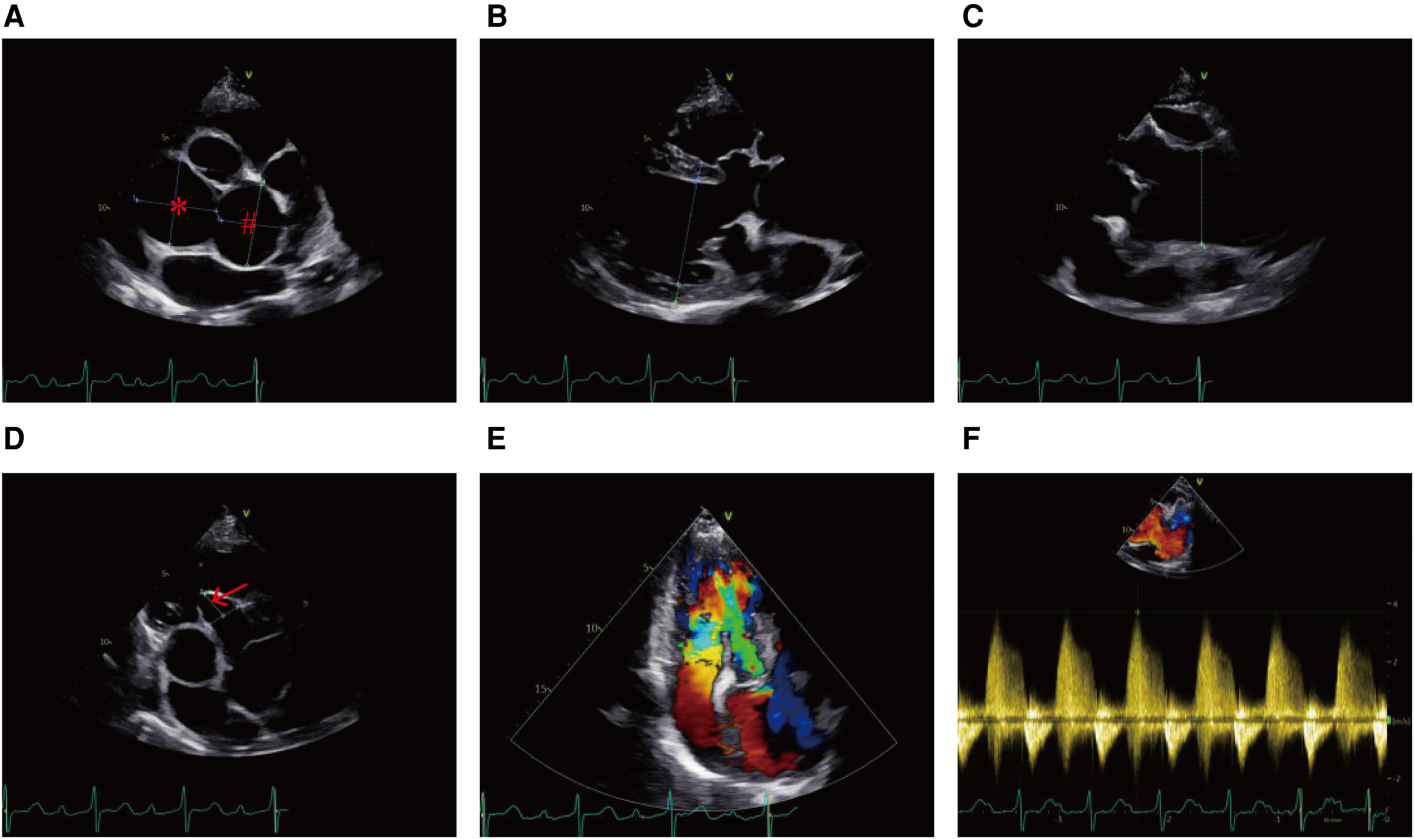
Echocardiogram showing (A) the unruptured left SVA (#) and non-coronary SVA (*), (B) enlarged left ventricle, (C) dilated aortic root, (D) ventricular septal membranous aneurysm (red arrow), (E) severe aortic regurgitation, and (F) right ventricular high pressure.
